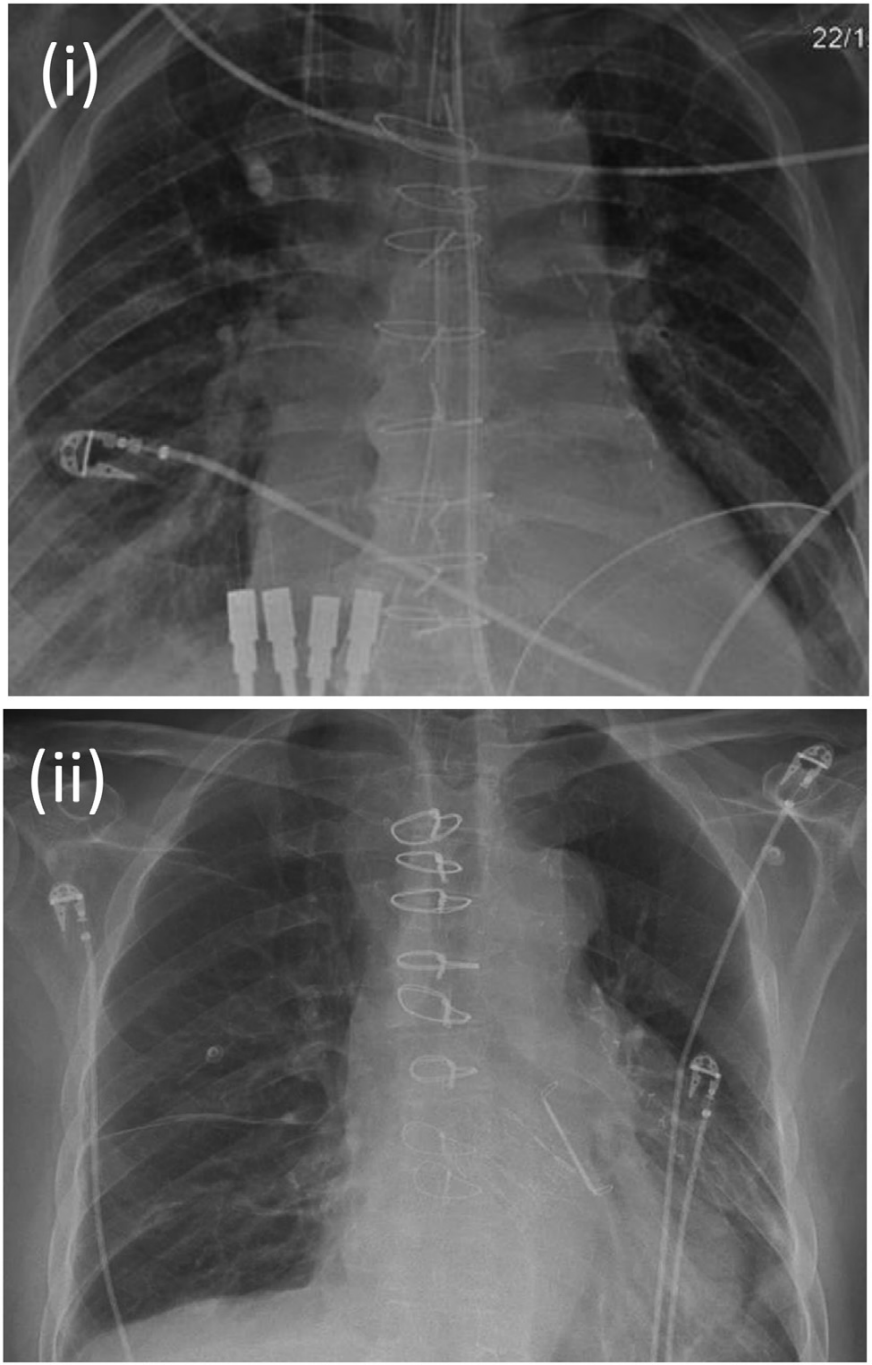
Example of (i) X-ray using sternal wire code (3 grafts, 1 from subclavian artery and 2 from aorta) and (ii) X-ray where code was not used.
